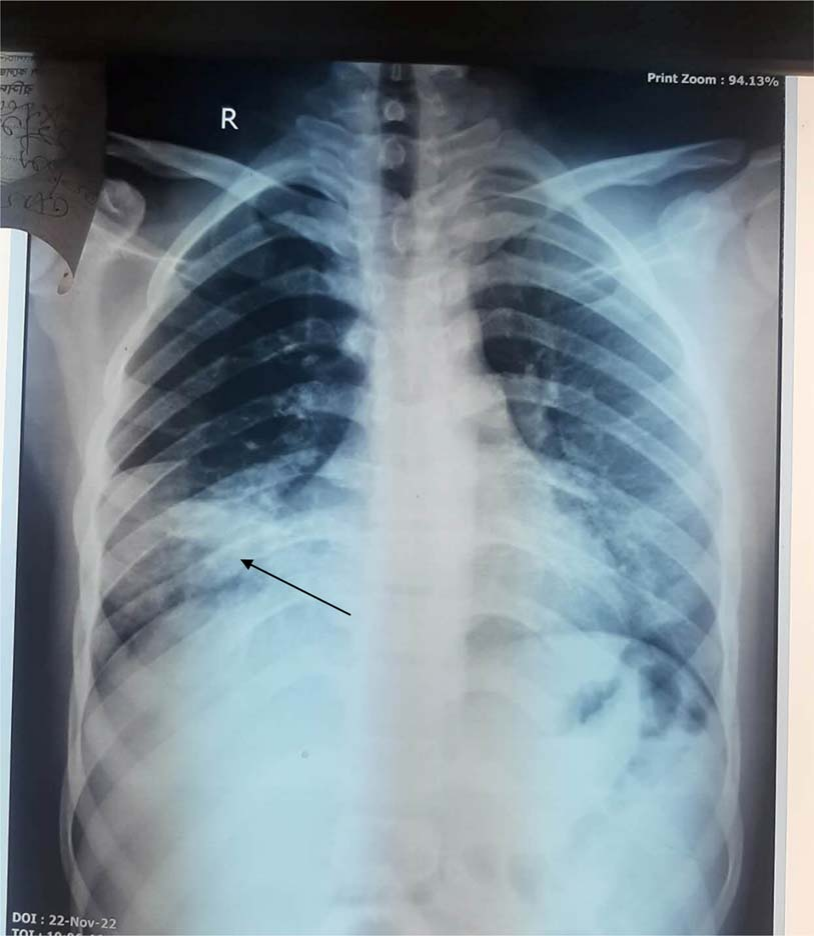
Chest X-ray (*P*/A view) showing bilateral patchy homogenous parenchymal lung opacities in right lower zone and left middle and lower zones.
